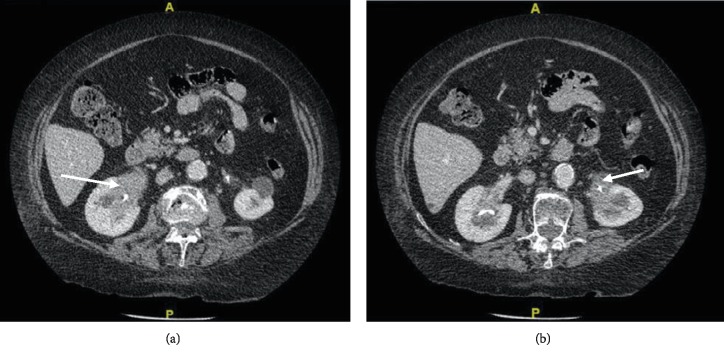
Injected computed tomography of the abdomen after bilateral ureteral stent insertion: *arrows* showing the infiltration of the right (a) and left (b) kidneys by a homogeneous tissue enclosing the pelvis, signs of retroperitoneal fibrosis. No characteristic “hairy kidney” appearance and no signs of aortic involvement.
